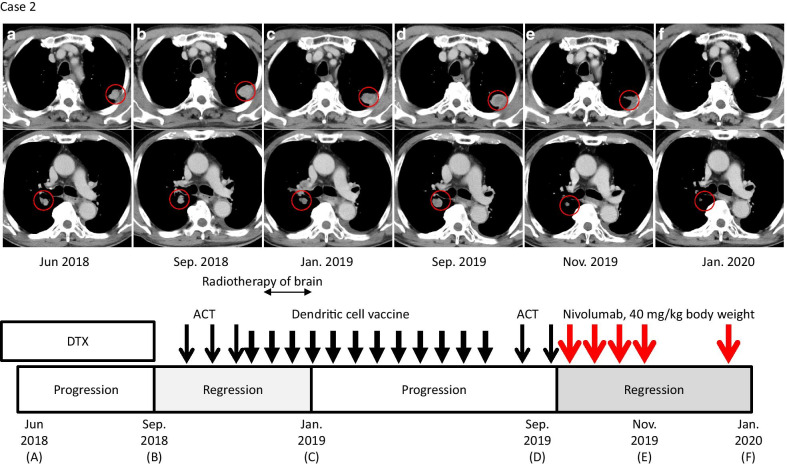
Clinical course of case 2. Axial CT images corresponding to the timelines of therapy and disease status. Red circles indicate the lung and hilar metastatic lesions. a (top) Status during administration of docetaxel (DTX). b Progression of lung metastasis before treatment with adoptive cell therapy (ACT). c Regression of lung metastasis after treatment with ACT and dendritic cell (DC) vaccine. d Progression of lung and hilar lymph node metastases before administration of nivolumab. e Regression of lung metastasis 8 weeks after immune checkpoint inhibitor (ICI) and ACT combination treatment. Responses were durable during maintenance therapy with the ICI (f). Narrow black arrows, bold black arrows, and red arrows indicate ACT, DC vaccine, and nivolumab administration, respectively (bottom)
